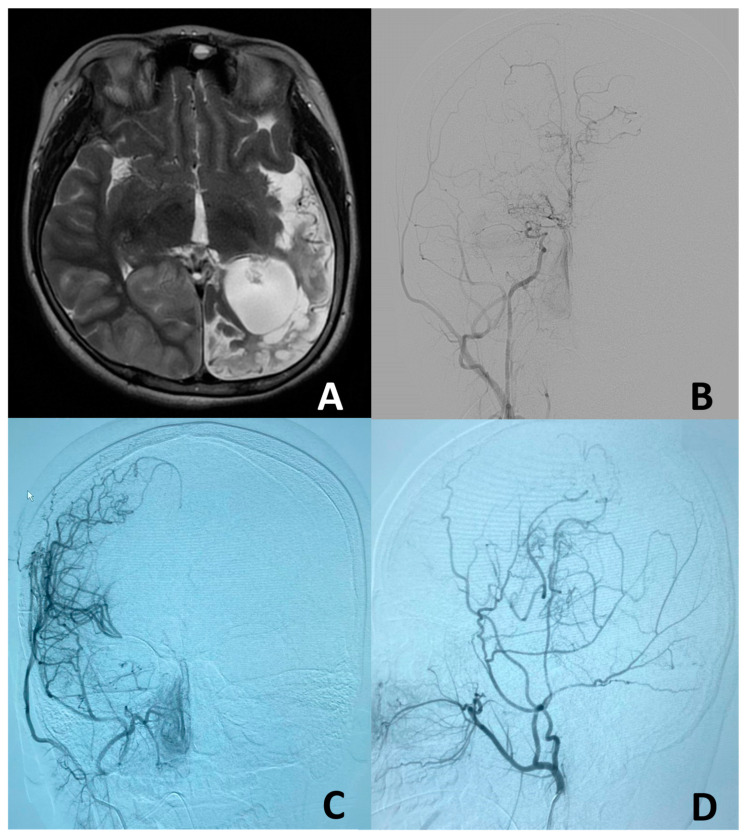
A 9-year-old girl with right-sided hemiparesis and prior left ICA territory infarction. (A) MRI demonstrating postischemic gliosis. (B) Preoperative DSA showing right terminal ICA occlusion consistent with Suzuki stage V moyamoya disease. Follow-up angiography at 14 months (C,D) demonstrates Matsushima grade B collateral formation after indirect revascularization.
